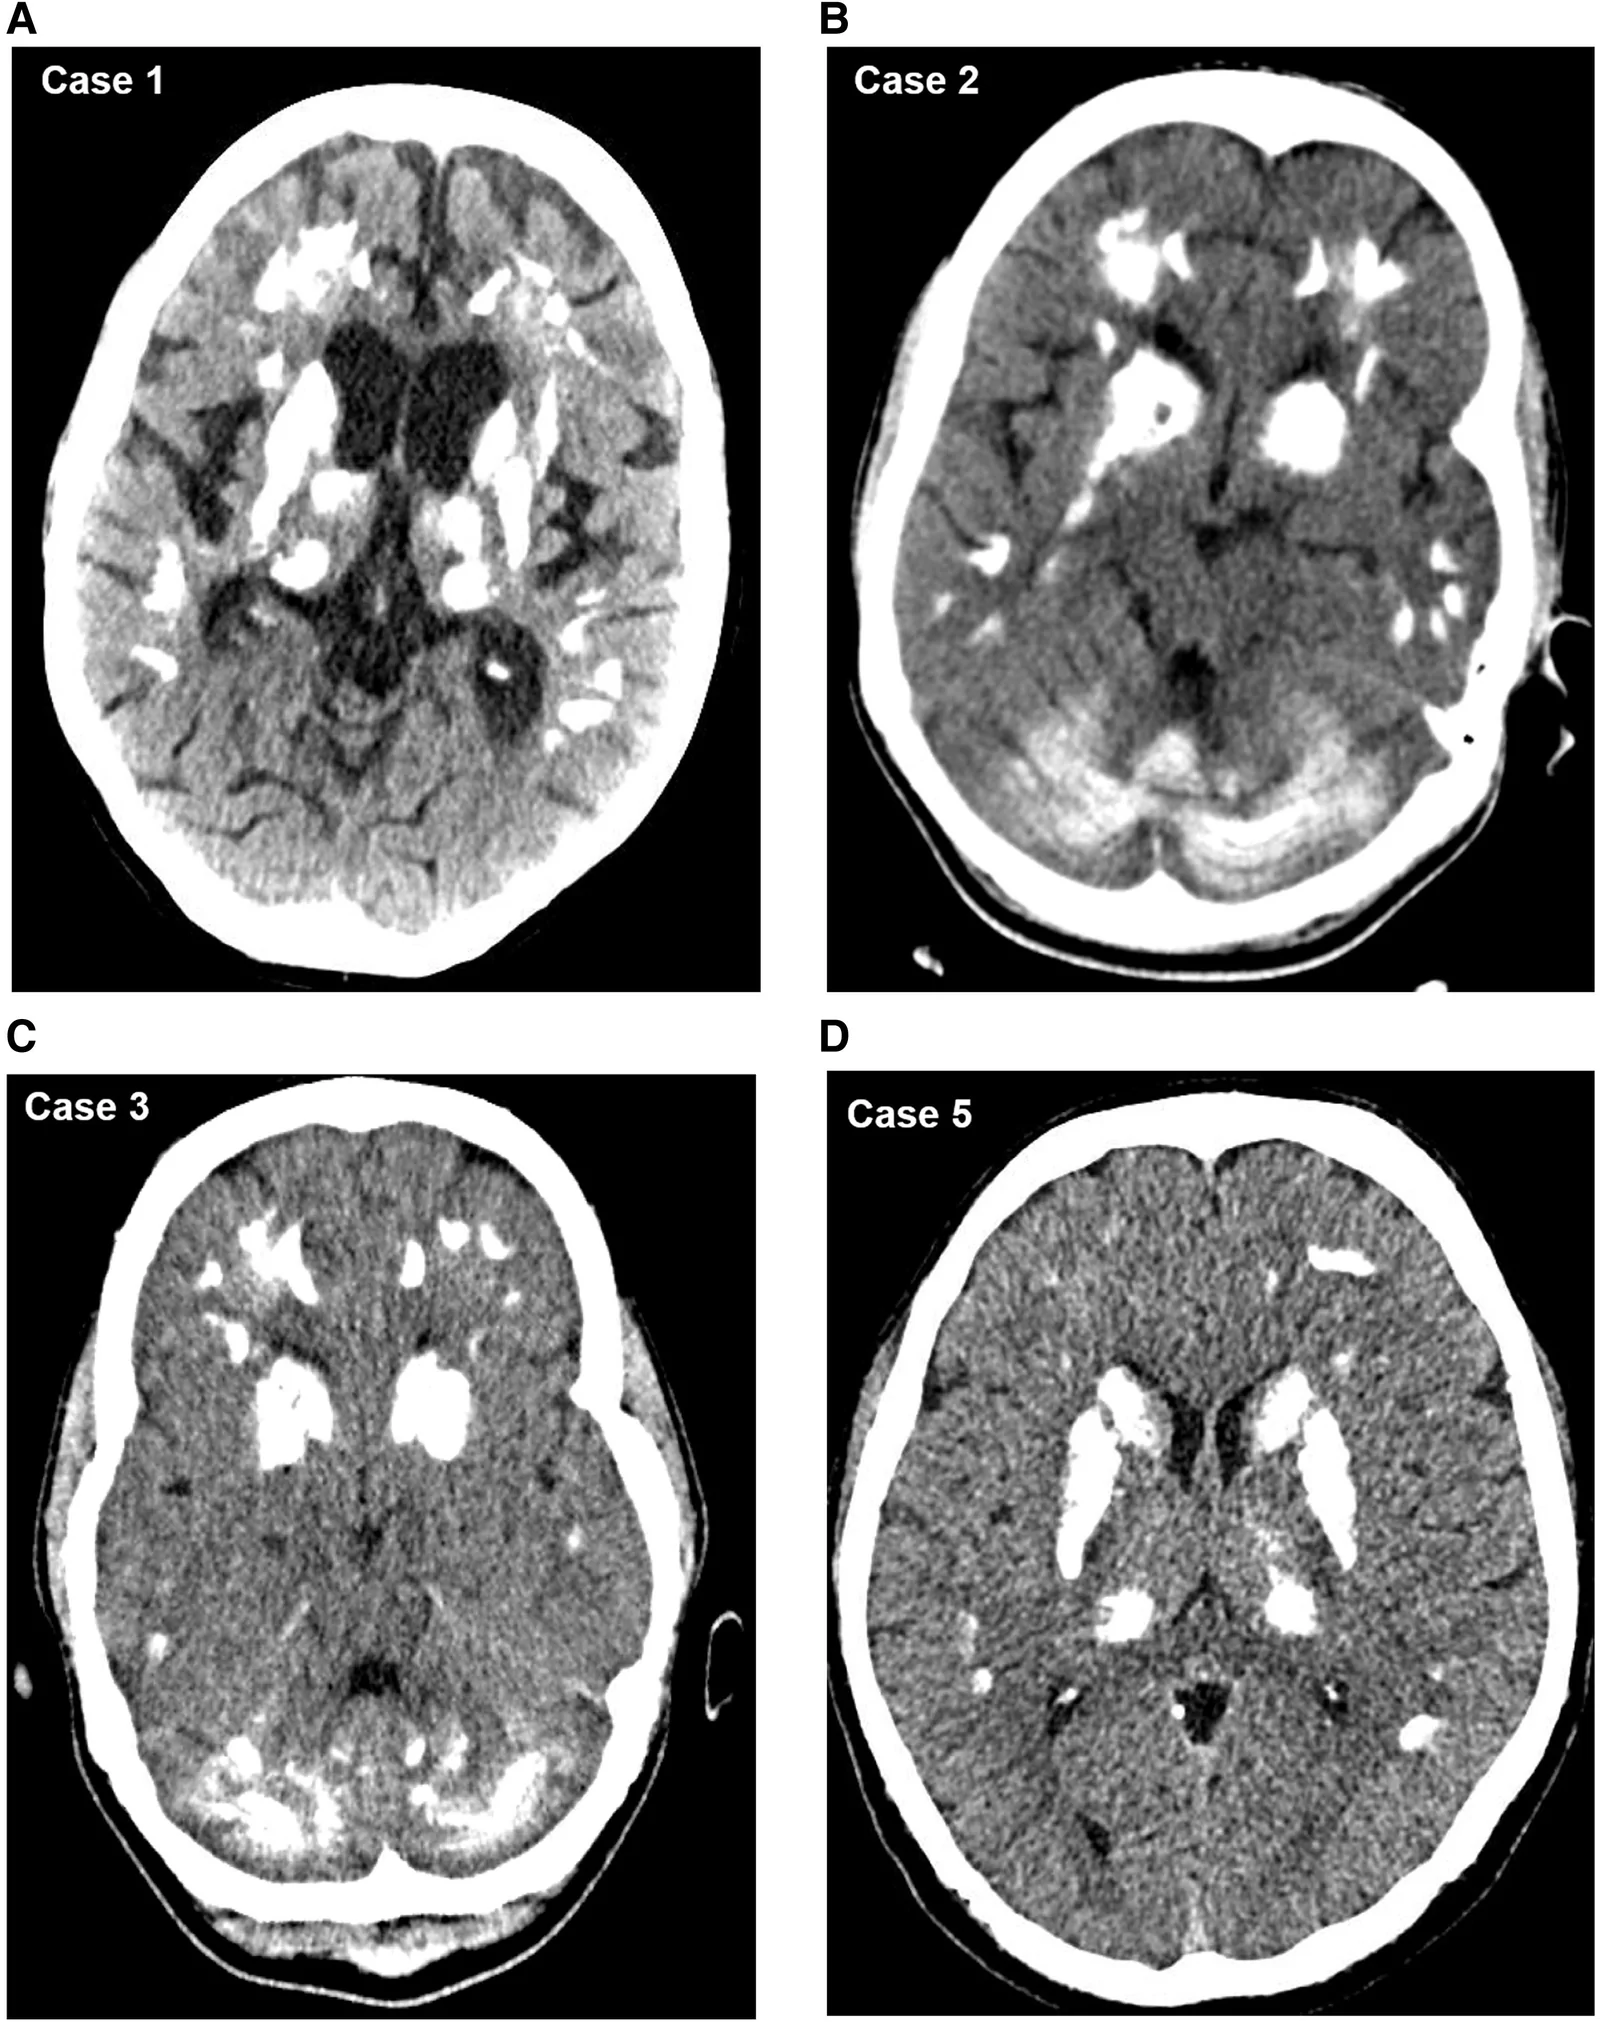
Intracranial calcification is a major phenotype of autosomal dominant hypocalcemia type 1 patients. Computed tomography imaging of brain (axial views) showing brain calcification in cases 1 to 3 (family 1) and 5 (family 3).
